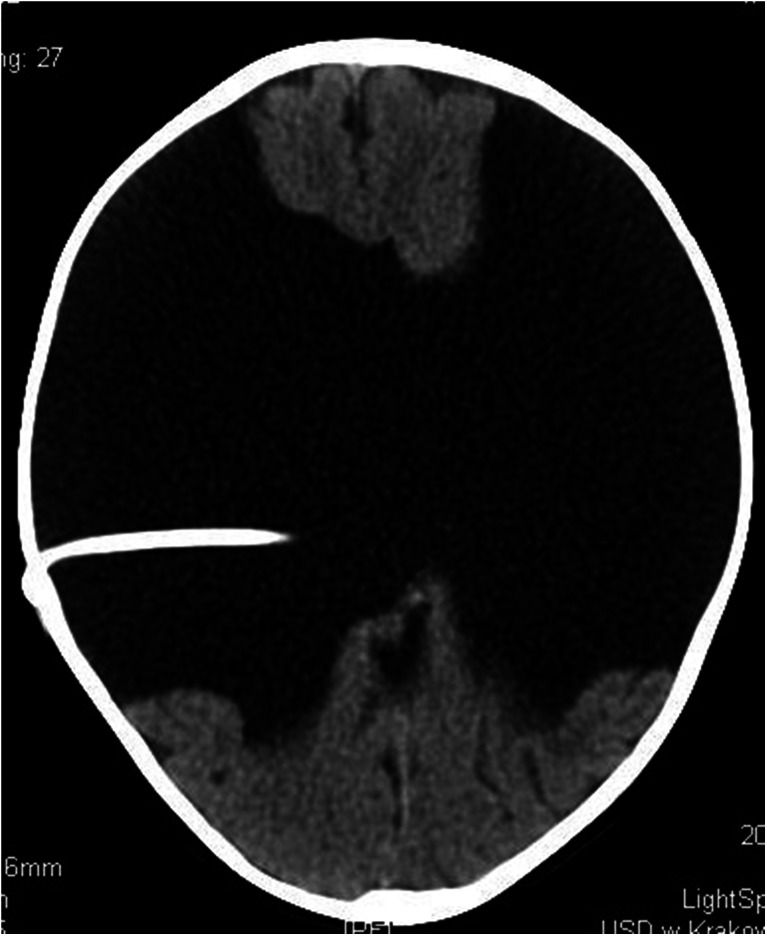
A follow-up scan 8 months following shunting. Persistent supratentorial fluid-filled spaces of a comparable size. The longitudinal fissure of the brain and brain sulci are seen more clearly as compared to the preoperative scan, what indicates decreased intracranial hypertension. Nonenhanced CT scan
